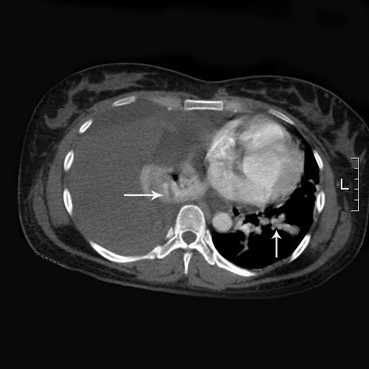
Sample figure title. Contrast-enhanced computed tomographic scan of the thorax shows blood in the right pleural space and compression atelectasis of the right lung. Note also the high-density nodules (arrow) located right lower lobe and left lower lobe, possibly indicative of the PAVMs.
